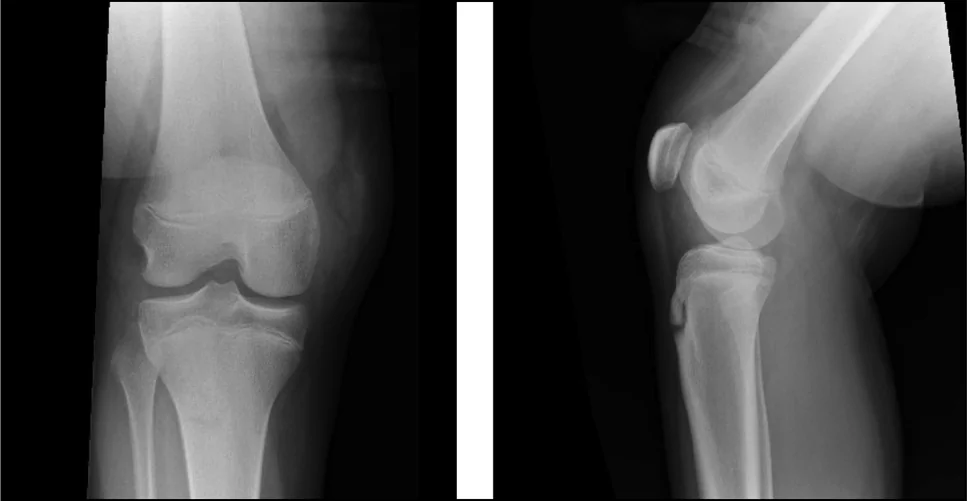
Anteroposterior and lateral radiographs of the right knee showing open distal femoral and proximal tibial physes.
